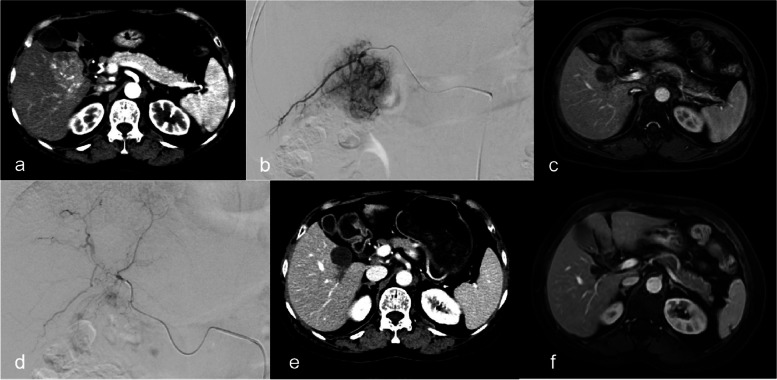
a. 66 years old, female patients, contrast enhanced CT cross sectional image demonstrating a HCC lesion in segment 6 and PVTT in the second order portal vein. b. the initial DEM-TACE angiogram demonstrate the feeding artery of tumor and PVTT. c. Contrast enhanced MRI 1 months after the initial DEM-TACE demonstrate the residual tumor. d. second DEM-TACE angiogram demonstrating the residual tumor. e. CT images acquired 1 months after the second DEM-TACE demonstrate no viable tumour and PVTT (radiologic response: CR). f. MRI images acquired more than 26 months after the second DEM-TACE demonstrate that the disappearance of PVTT and the patent portal vein
